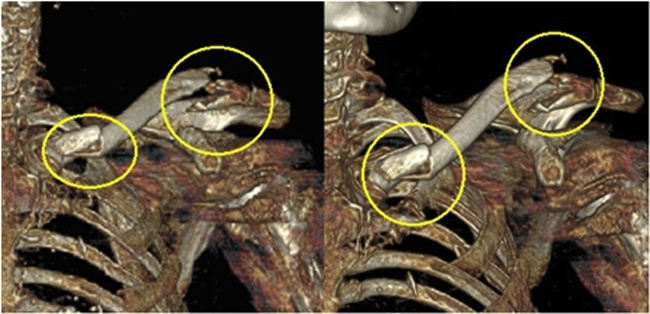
CT scan 3D reconstruction of the left clavicle showing a bipolar clavicular displaced fracture.
